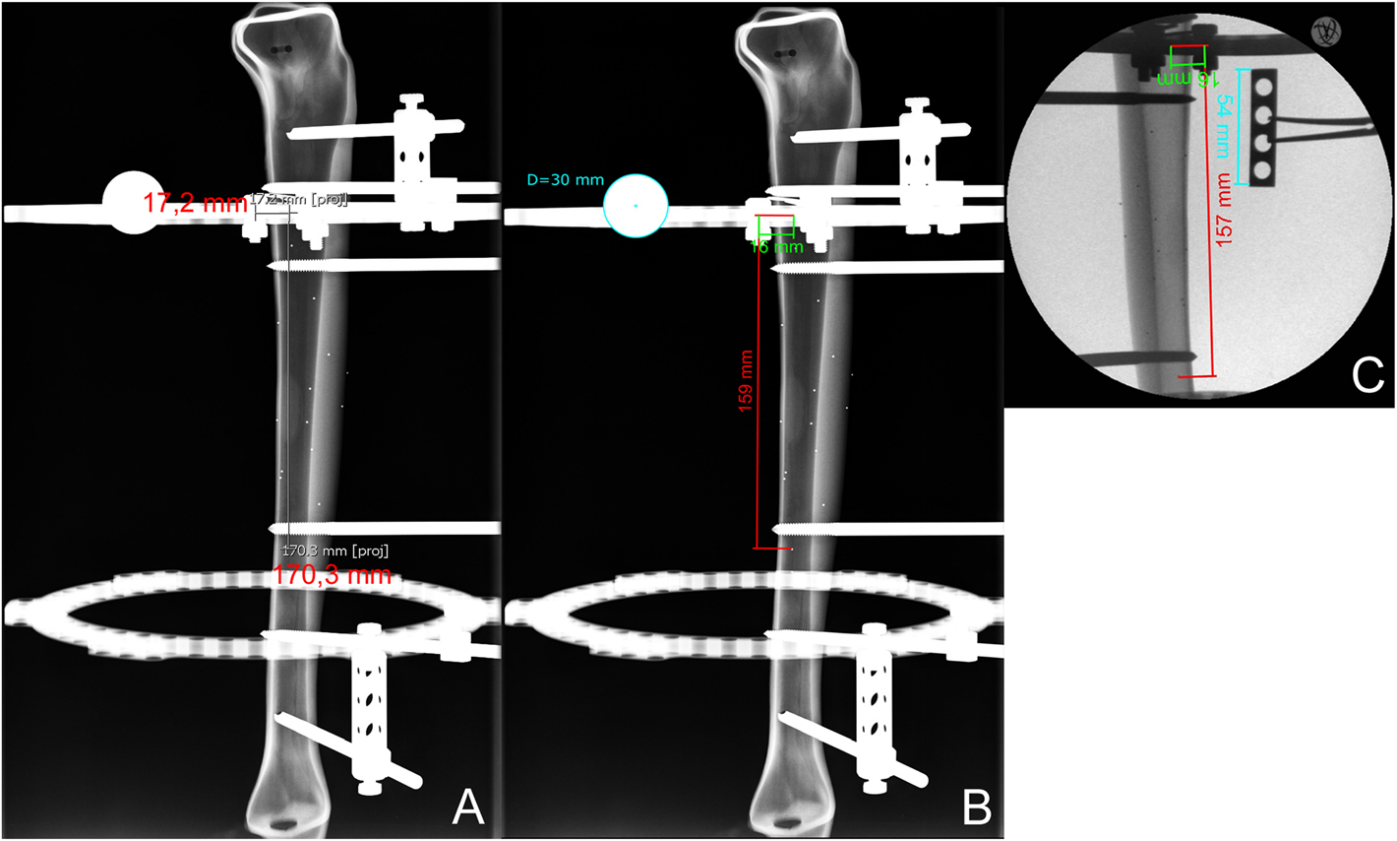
Lateral view for axial and anteroposterior MP measurements showing the superimposed bolts on an X-ray image without (a, method A) and with (b, method B) calibration as well as on a fluoroscopic intensifier image (c, method C); the axial MP on X-ray image a (170 mm) shows high deviation compared to those on calibrated X-ray image b (159 mm) and fluoroscopic image c (157 mm), whereas the anteroposterior MP shows only a minor deviation of 1 mm (a: 17 mm, b and c: 16 mm)
